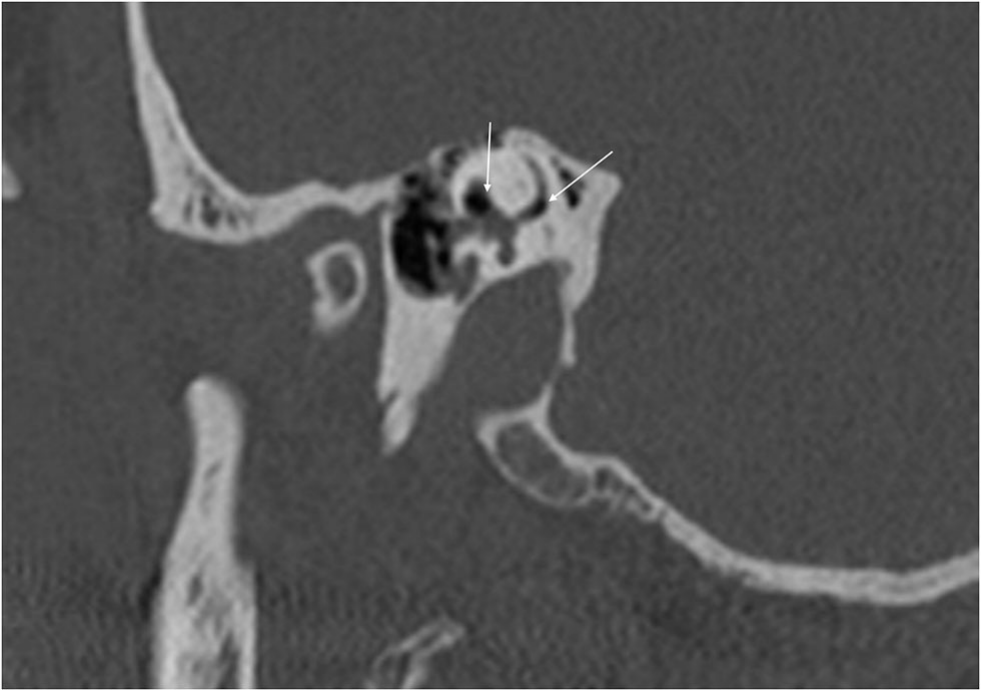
Sagittal CT of temporal bone demonstrating air in the vestibule and the crus communs (arrows) in a patient with perilymph fistula.
